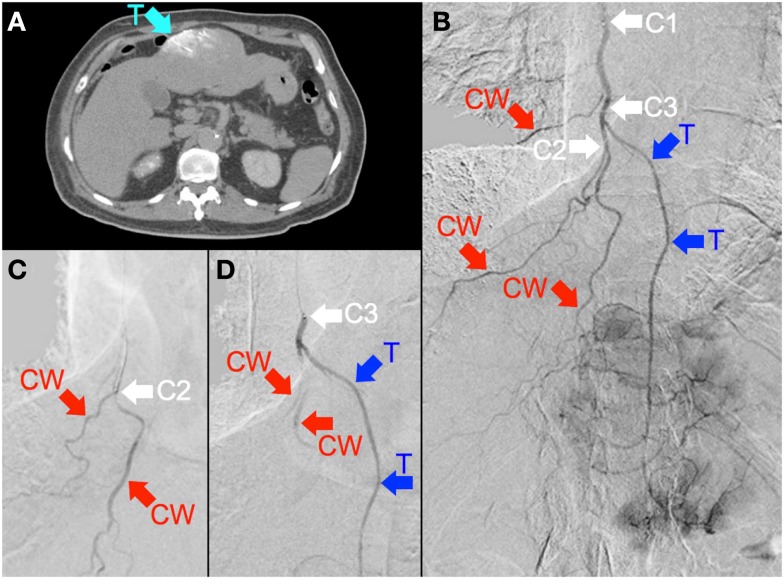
(A) Multifocal hepatocellular carcinoma with a large segment III tumor. Catheter-directed CT hepatic angiogram of the right internal mammary artery (RIMA) demonstrates blood supply to one-third of the large segment III tumor (“T”). (B) Digital subtraction angiogram (DSA) of the RIMA demonstrates the target arterial tree, with three major branches supplying the right lower anterior chest wall (“CW”) and a terminal branch supplying tumor (“T”). The tumor blush is seen distally. The catheter tip was positioned at the proximal RIMA (“C1”) for ^99m^Tc MAA injection. (C) To minimize non-target flow of ^90^Y resin microspheres to the chest wall, the catheter tip was advanced to the origin of two chest wall arteries (“C2”), where prophylactic bland embolization with gel foam slurry was performed until vascular stasis; coil embolization at this position was technically not possible due to vessel tortuosity. (D) The catheter tip was then pulled back slightly (“C3”), proximal to the origin of the tumor branch (“T”). RE was performed at this position with intermittent checks by DSA to ensure good forward flow and no reflux of contrast.
